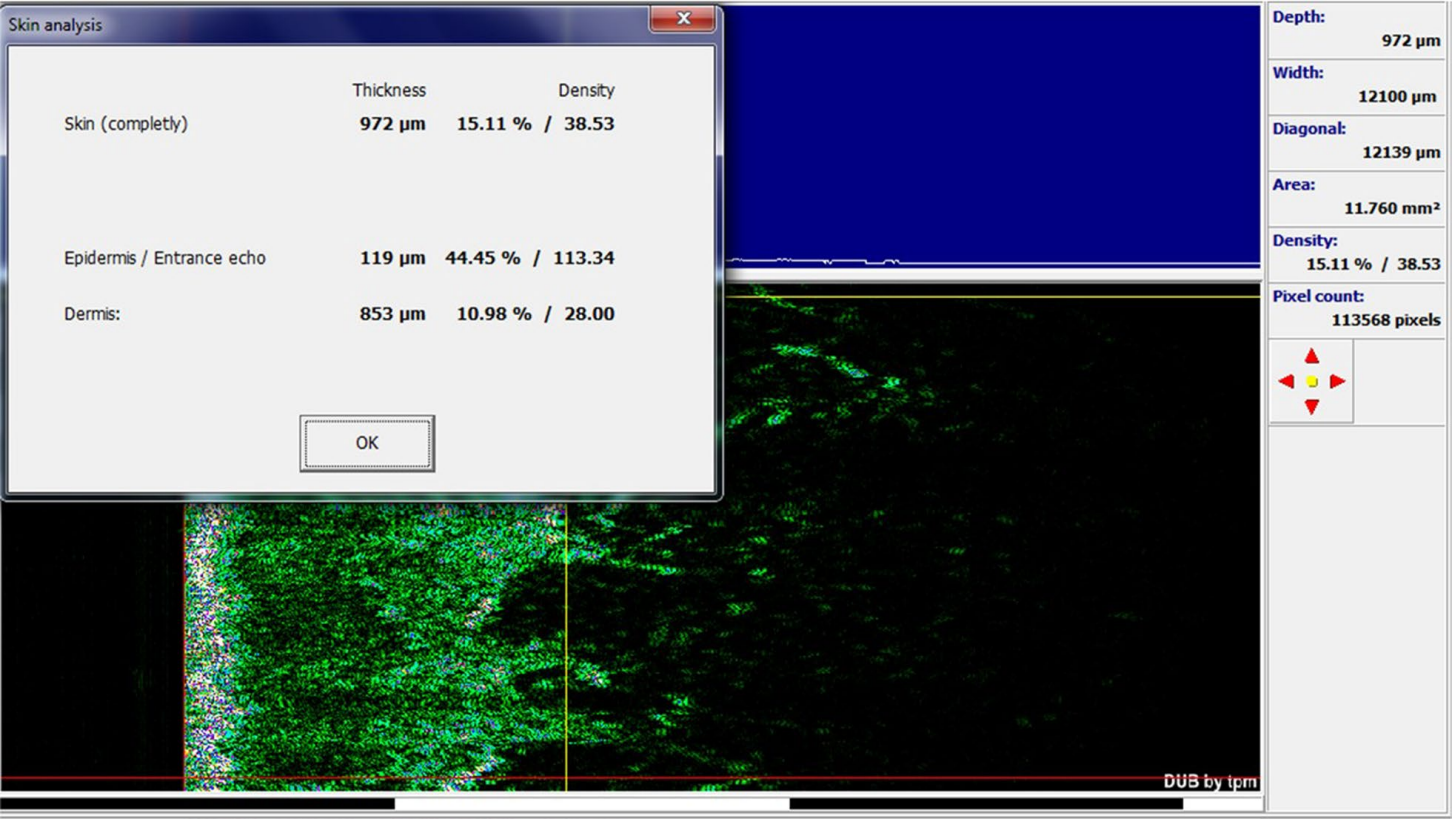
Sonographic findings before intervention with fractional CO_2_ laser and SVF
